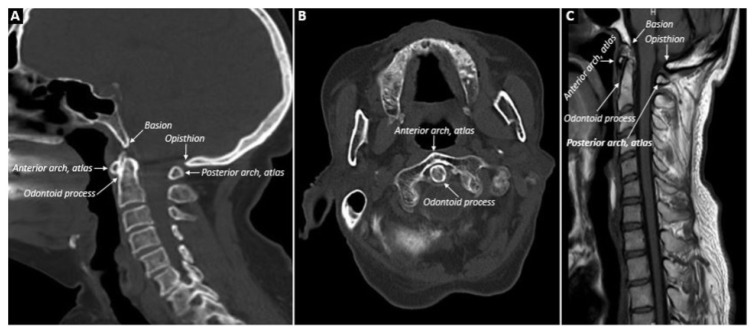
Normal anatomy of the cervical region is shown in A, B and C, A: sagittal CT; B: axial CT; C: sagittal MRI.
